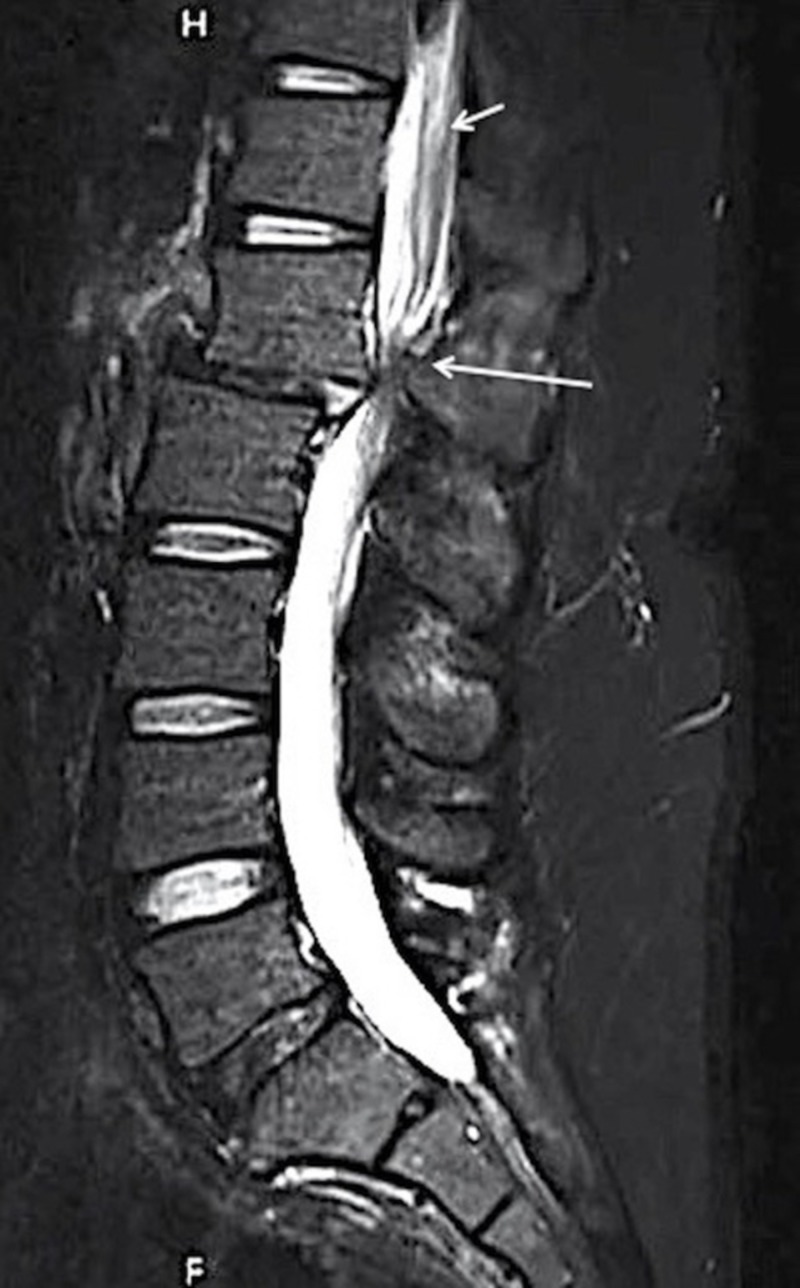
MRI L spine post mobilization L1-2 distraction injury and severe canal compromise from ligamentous damage (long white arrow). Extensive conus and cord T2 hyperintensity is shown (short white arrow), suggesting vascular injury from severe venous compression at the L1-2 level. MRI: magnetic resonance imaging
